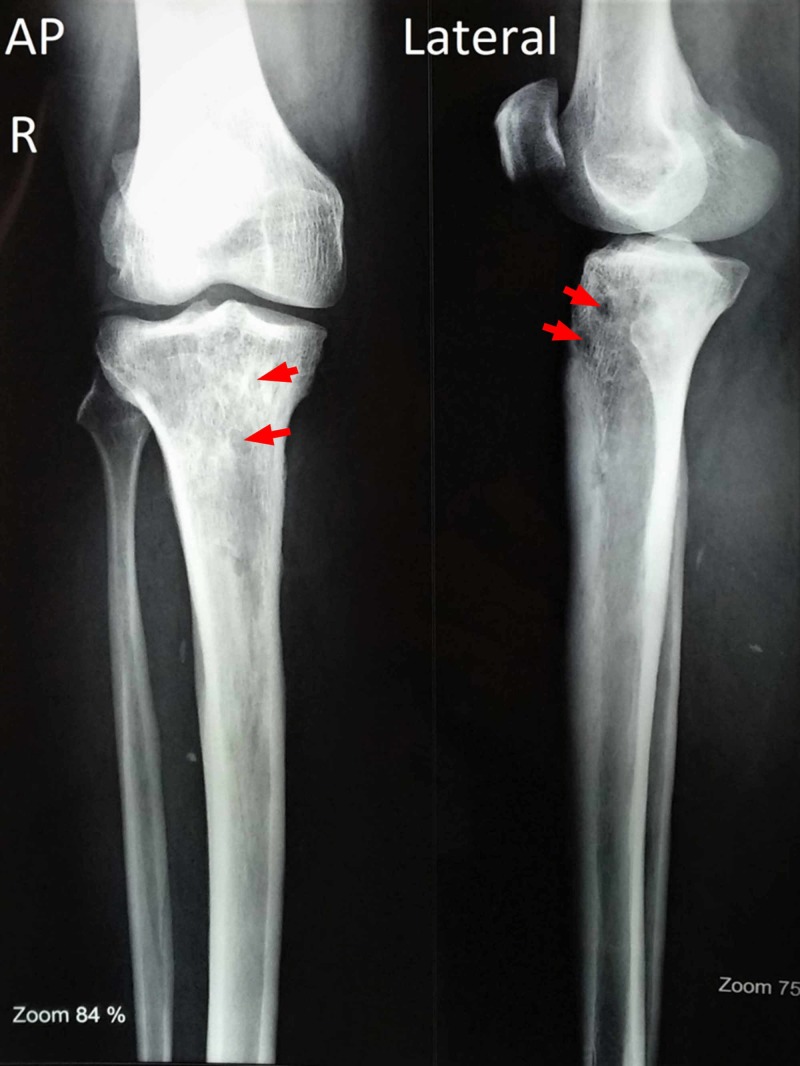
X-ray of right leg taken six months postoperatively showing few lytic lesions (red arrows) in the proximal tibia.
